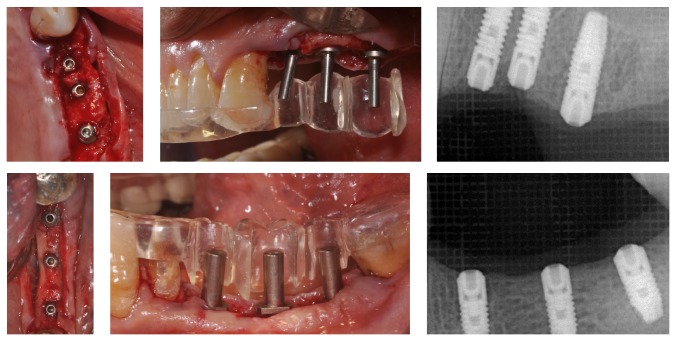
Type 3 prosthetically guided implant positioning, surgical and radiographic view.
